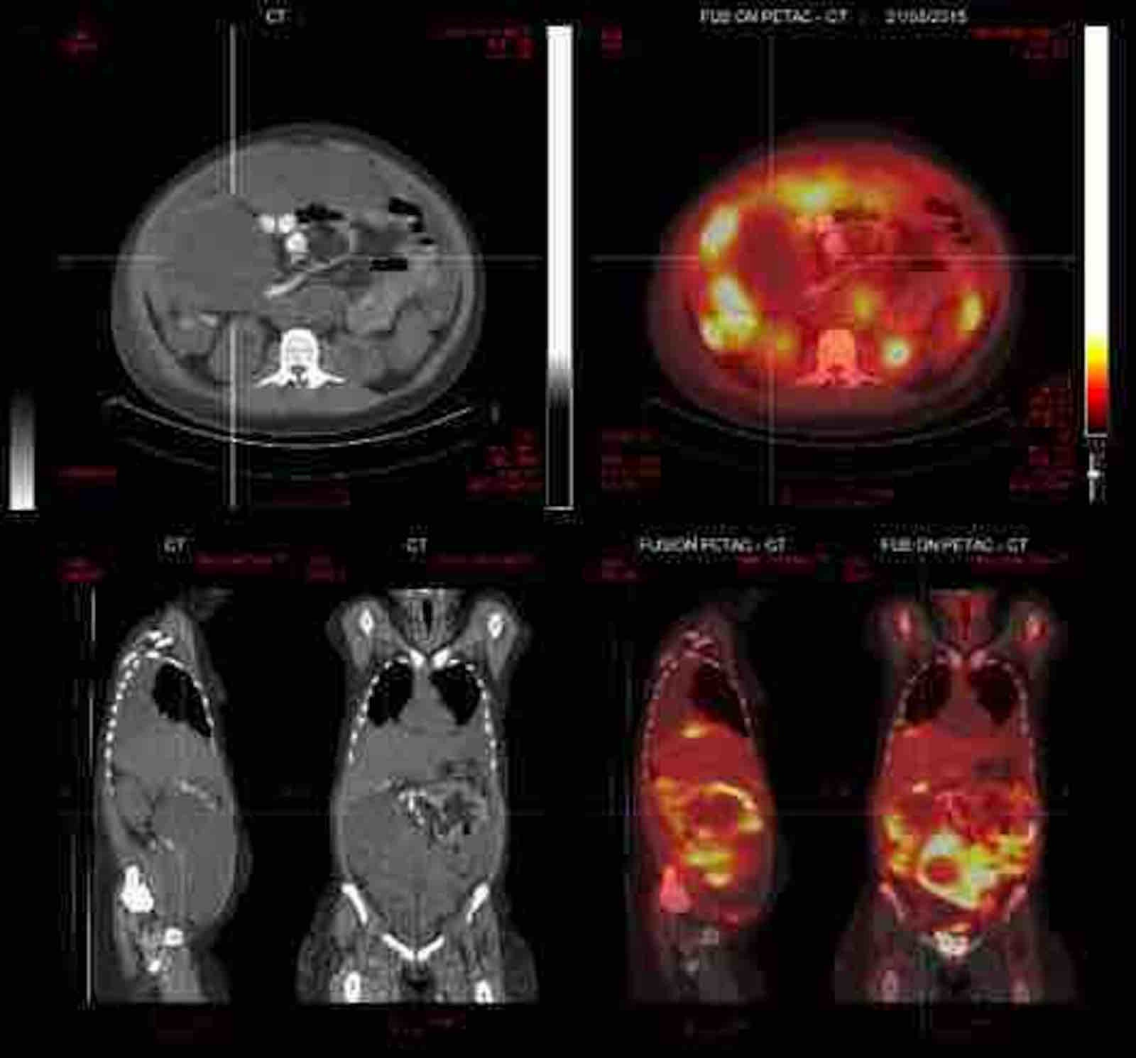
Staging PET-CT scan. PET-scan before the first cycle of chemotherapy showing multiple areas of increased metabolic activity in the abdomen and pelvis (maximum SUV 11.6) along with bilateral pleural effusions.
